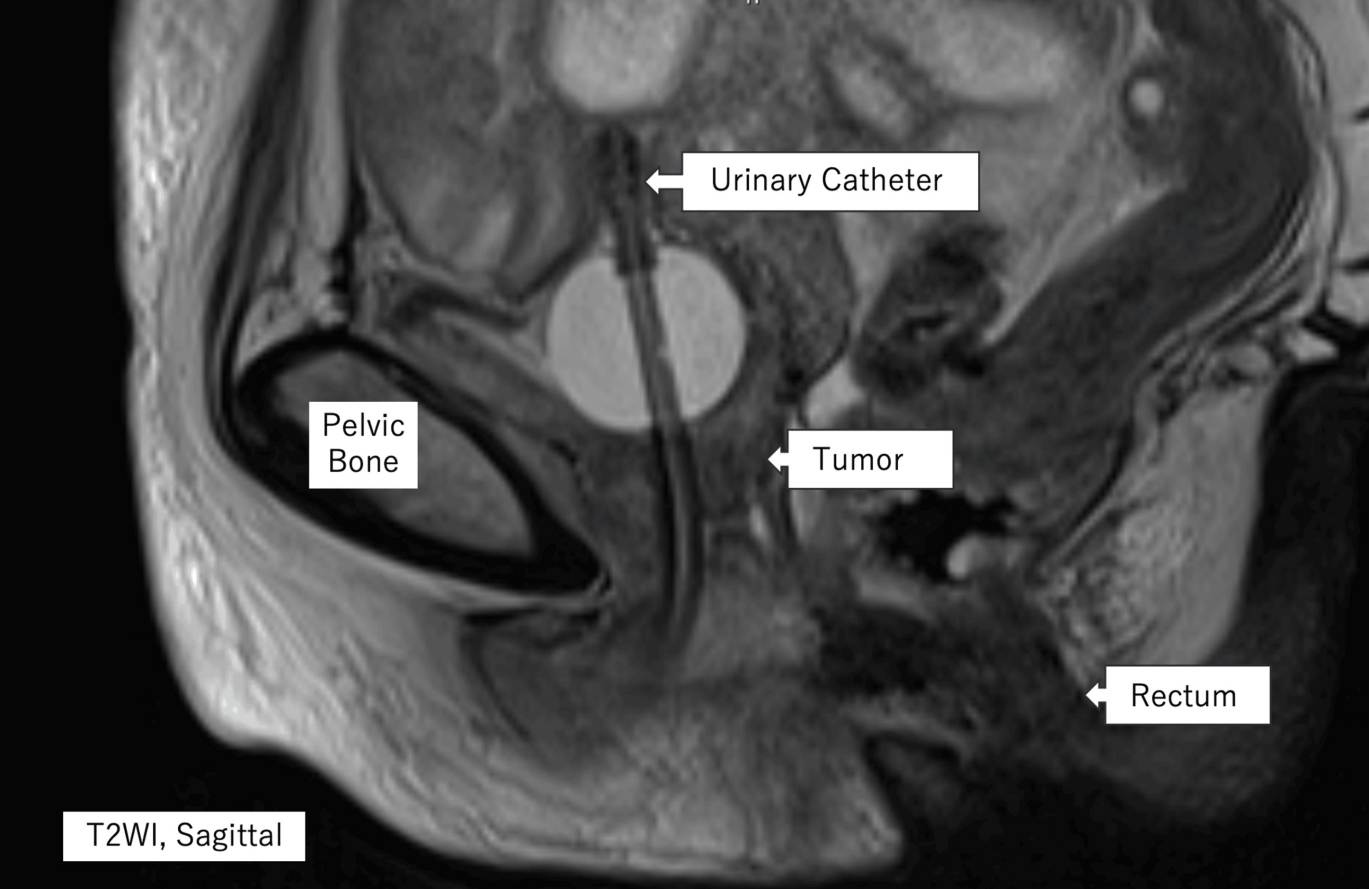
Pelvic MRI after radiotherapy A T2-weighted sagittal image of the pelvis taken post-radiotherapy shows marked tumor regression.
